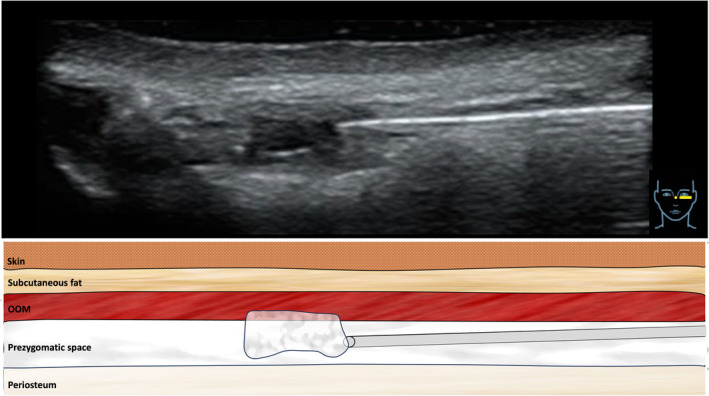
Ultrasound image showing the placement of soft tissue fillers in the prezygomatic space. The ultrasound transducer was placed in a horizontal fashion.
